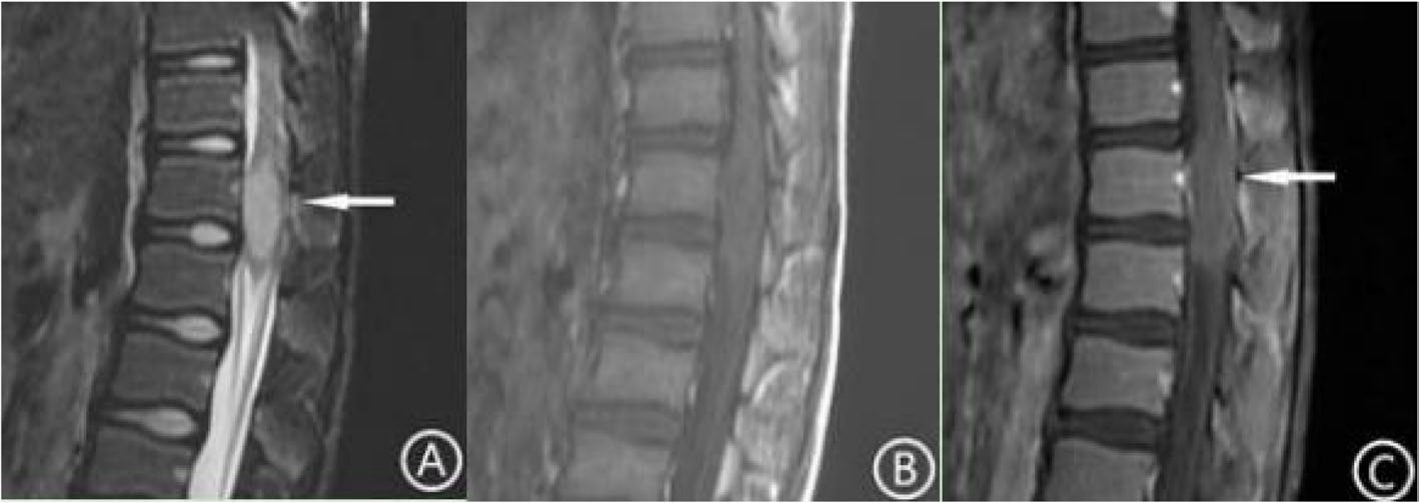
MR images of the thoracic vertebrae in patients with Diffuse midline glioma, H3K27-altered. The tumor was located in the conus medullaris. The internal signal was uniform, T2WI showed a high signal (A), and T1WI showed a low signal (B). There was no cystic necrosis inside the lesion (A, arrow) and no edema around the tumor. After enhancement, the tumor showed uniform mild enhancement (C, arrow)
